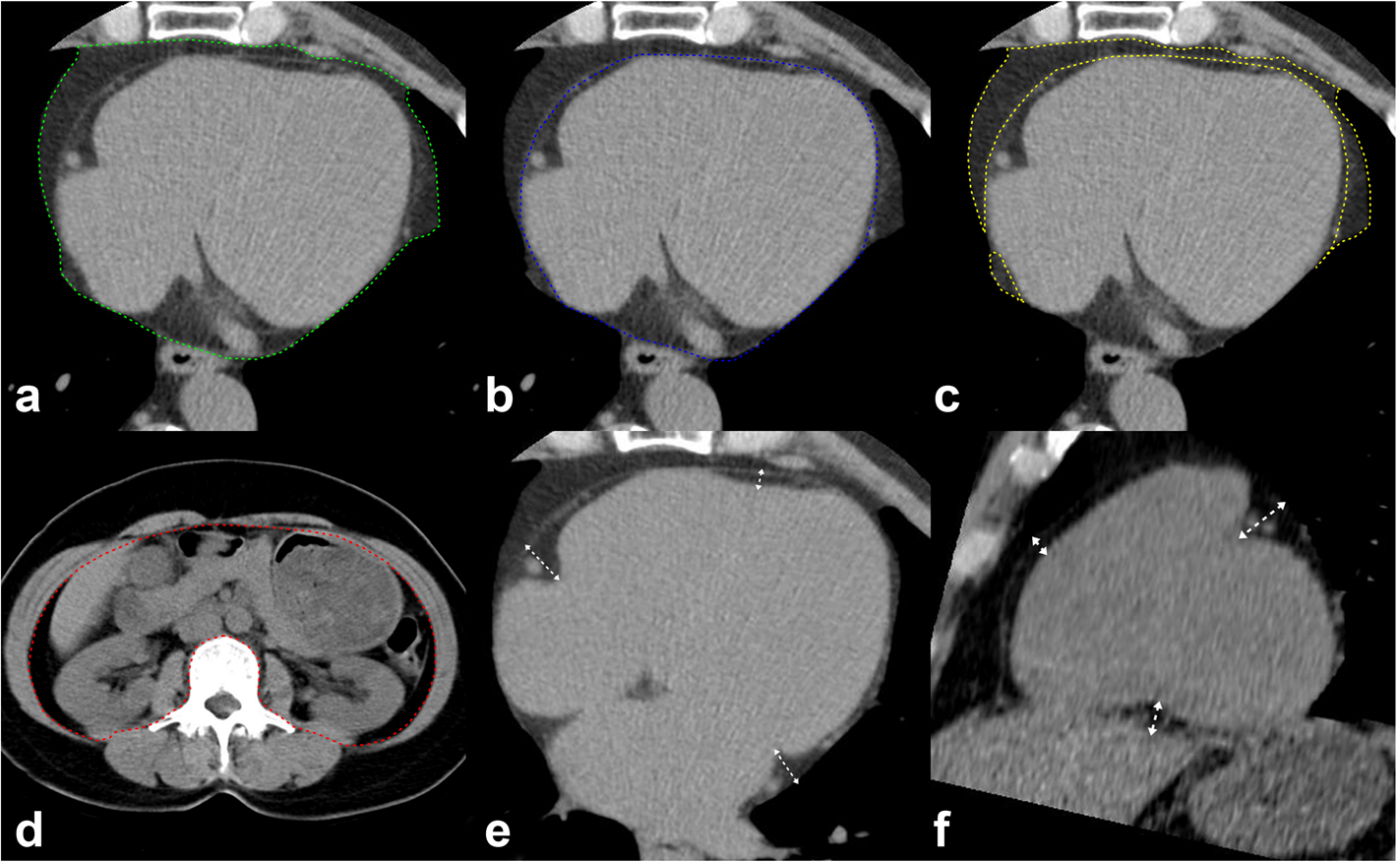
Quantification of different abdominal and cardiac fat deposits by CT with a threshold of −200 to −50 HU on a 3-dimensional workstation. a Measurement of total pericardial fat volume by manual tracing (*green boundary*) of the area of the pericardial fat from the left main pulmonary artery level to the left ventricular apex. b Measurement of total volume of EAT was performed on axial images by manual tracing (*blue boundary*) of the parietal pericardium from the left main pulmonary artery level to the left ventricular apex. c Total volume of PAT (*yellow boundary*) = total pericardial fat volume (PAT + EAT) – total EAT volume. d Measurement of total volume of AVAT was performed on axial images by manual tracing (*red boundary*) of the parietal peritoneum from L2 to L5 levels. e Regional EAT thickness was assessed as the distance from myocardium in the orthogonal direction to visceral epicardium on the horizontal long-axis plane at the left AVG, right AVG, and anterior IVG. f On the parasternal short-axis plane, regional EAT thickness was measured at superior IVG, inferior IVG, and right ventricular (RV) free wall. Double-headed arrows indicate the measurements of the EAT thickness. HU = Hounsfield units; EAT = epicardial adipose tissue; PAT = paracardial adipose tissue; AVAT = abdominal visceral adipose tissue; AVG = atrioventricular groove; IVG = interventricular groove; RV = right ventricle
